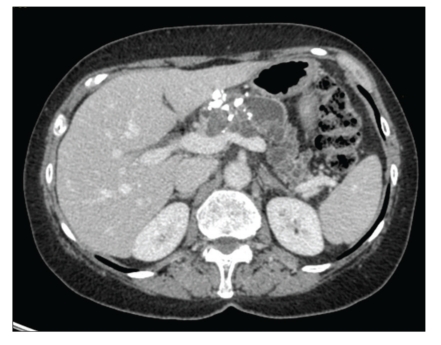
Preoperative computed tomography: presence of a well-defined multiloculated cystic mass in the body and tail of the pancreas with central calcifications.
